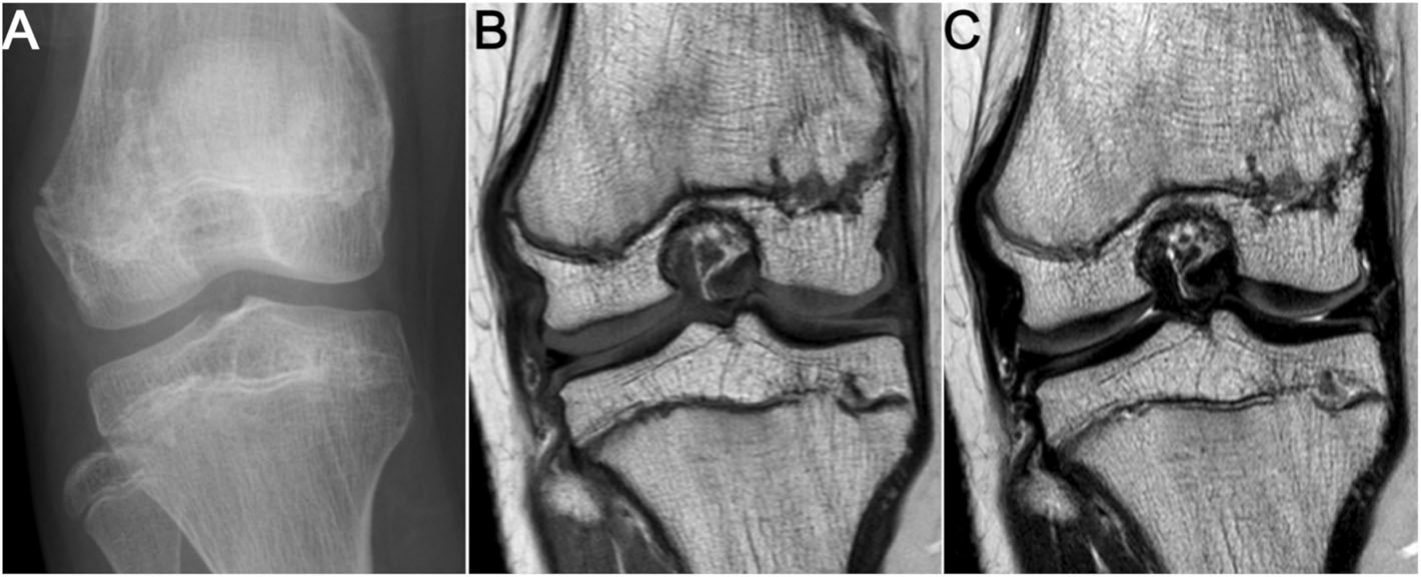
Close-up view of right distal femoral and proximal tibial physeal arrest. a Anteroposterior radiograph of right knee joint. b, c Coronal T1-weighted spin-echo (b) and T2-weighted spin-echo (c) MR images
